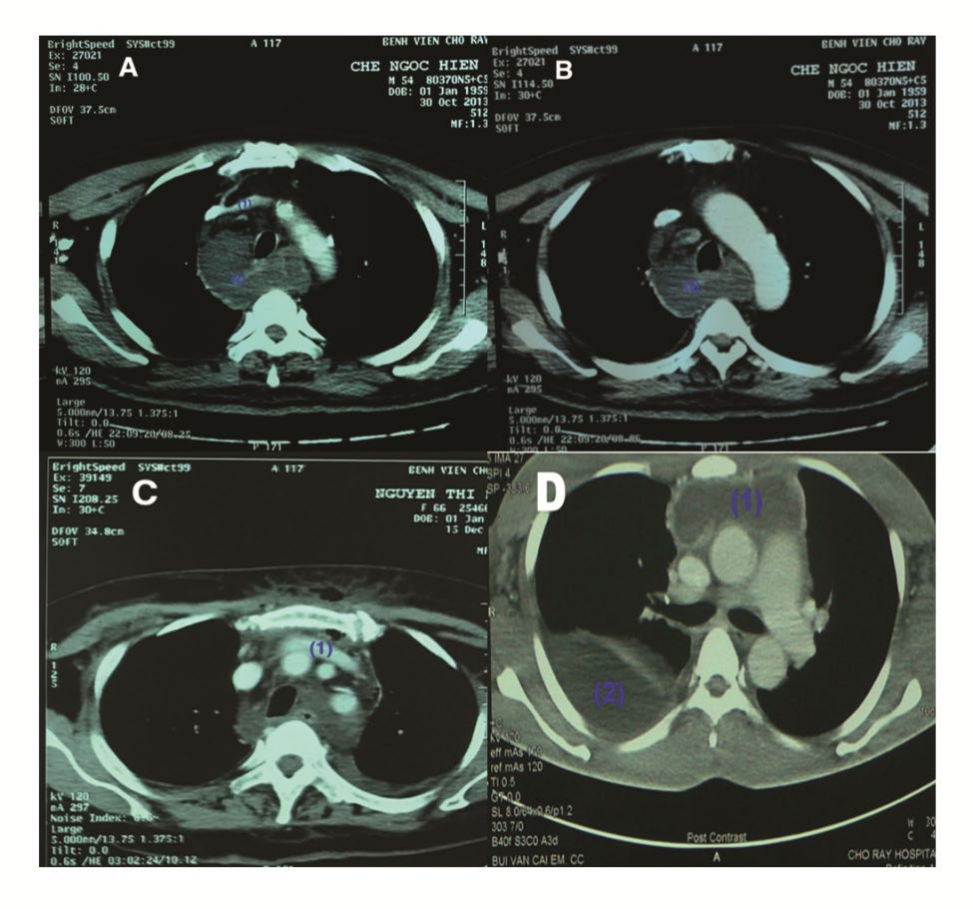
Obtained example computed tomography (CT) Scan results for the patients. (A) The infectious fluid (2) surrounding the trachea spread below the innominate vein (1). (B) The infection (2) is in the upper mediastinum. (C) The infection spread just to the level of the innominate vein (1). (D) the infection (1) spread to the lower mediastinum (carina level) plus right pleural effusion (2)
